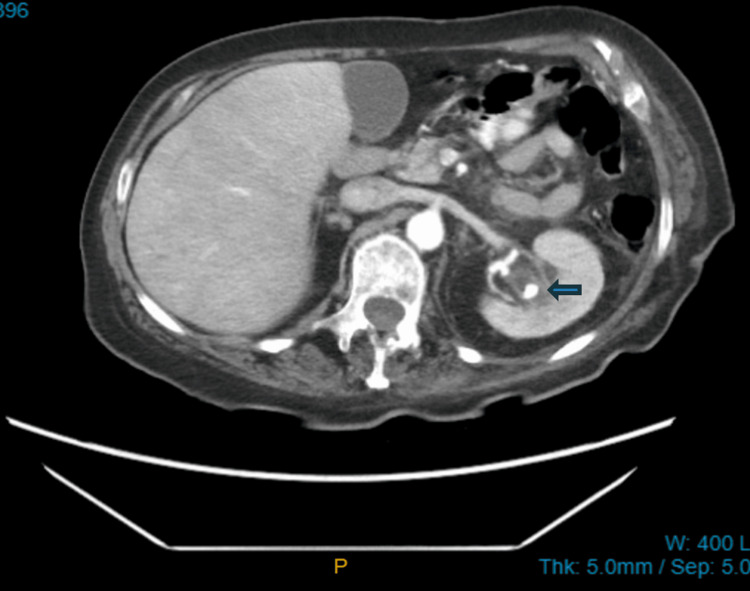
Axial view CT scan abdomen and pelvis showing left-sided nephrolithiasis The blue arrow demonstrates a large calcified stone measuring 11 × 11 × 10.4 cm.
